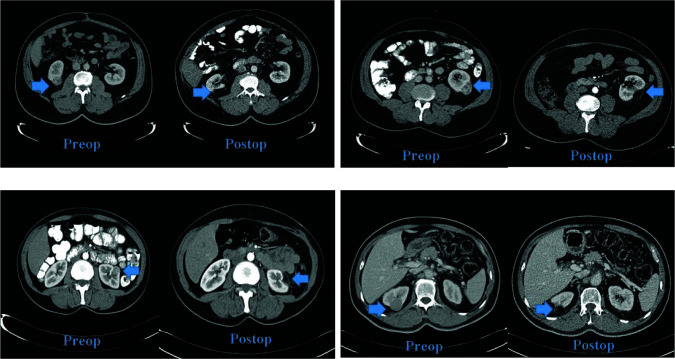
Image of postsurgical fatty tumor through contrast abdomen computed tomography.
